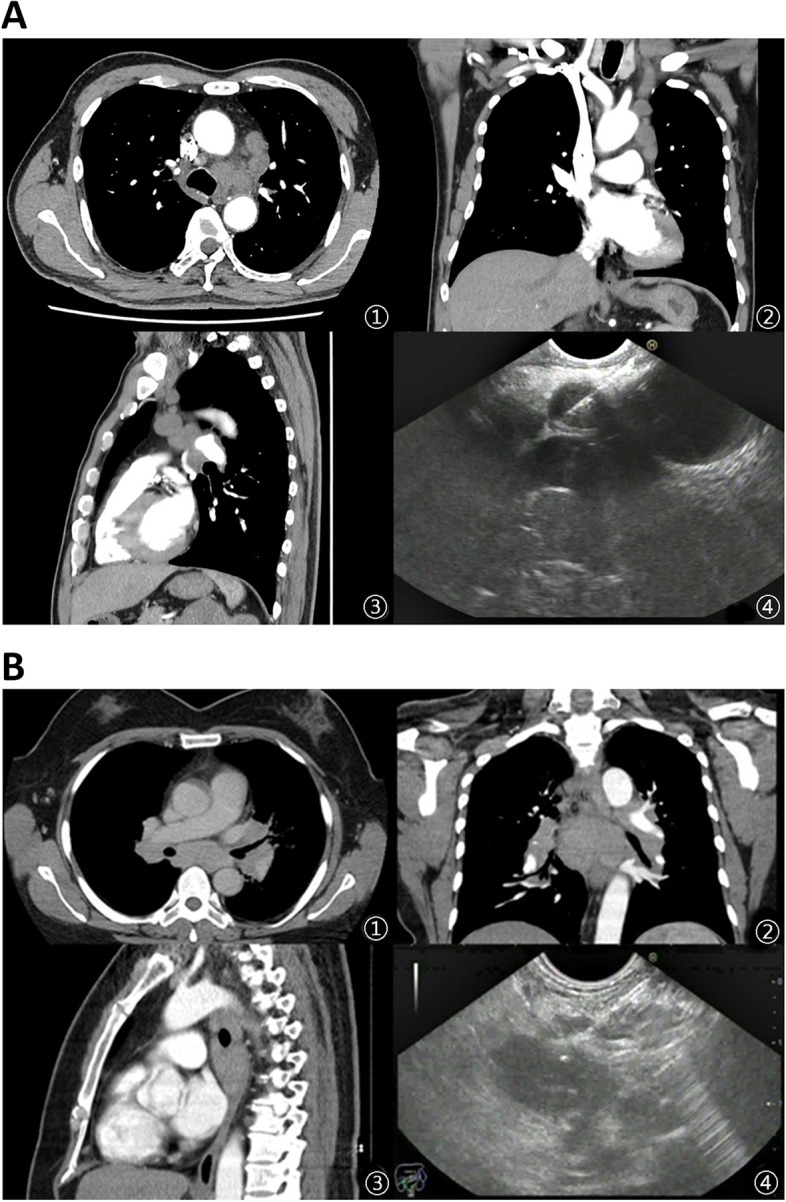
a A 56-year-old male patient. ①②③ Subcarinal lymph nodes are shown on CT scan. ④ Multiple round shaped lymph nodes were found in station 5 by EUS and EUS-FNA was performed. CT: computed tomography; EUS-FNA: endoscopic ultrasound-guided fine needle aspiration. b A 42-year-old female patient. ①②③ Left paratracheal lymph nodes are found on CT scan. ④ Oval hypoechoic lymph node was seen in station 4L by EUS and EUS-FNA was performed. CT: computed tomography; EUS-FNA: endoscopic ultrasound-guided fine needle aspiration
